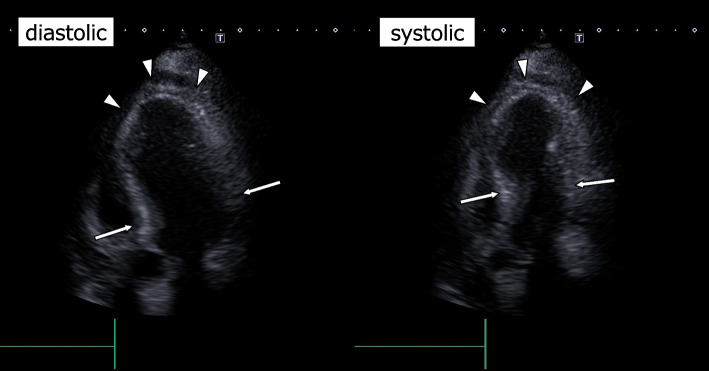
The postoperative transthoracic echocardiography of apical four chamber view. The echocardiography revealed apical hypokinesis (white arrowheads) and basal hyperkinesis (white arrows) of the left ventricular wall
